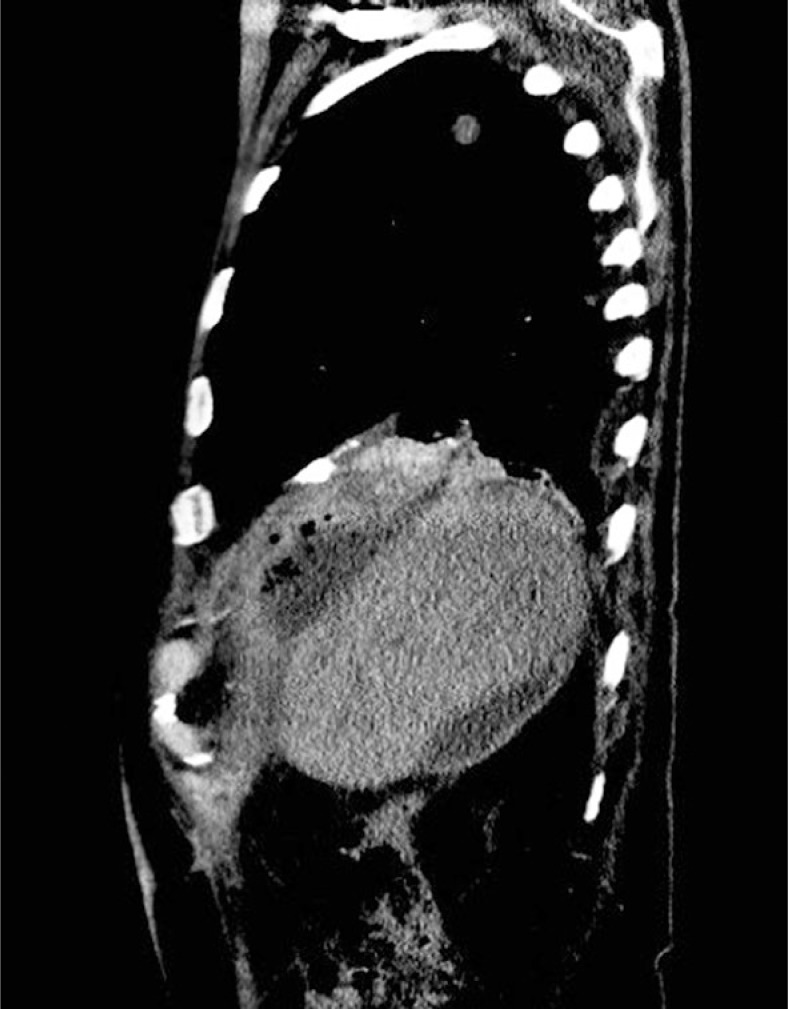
Bronchobiliary fistula (BBF) demonstrated by contrast enhanced CT.
